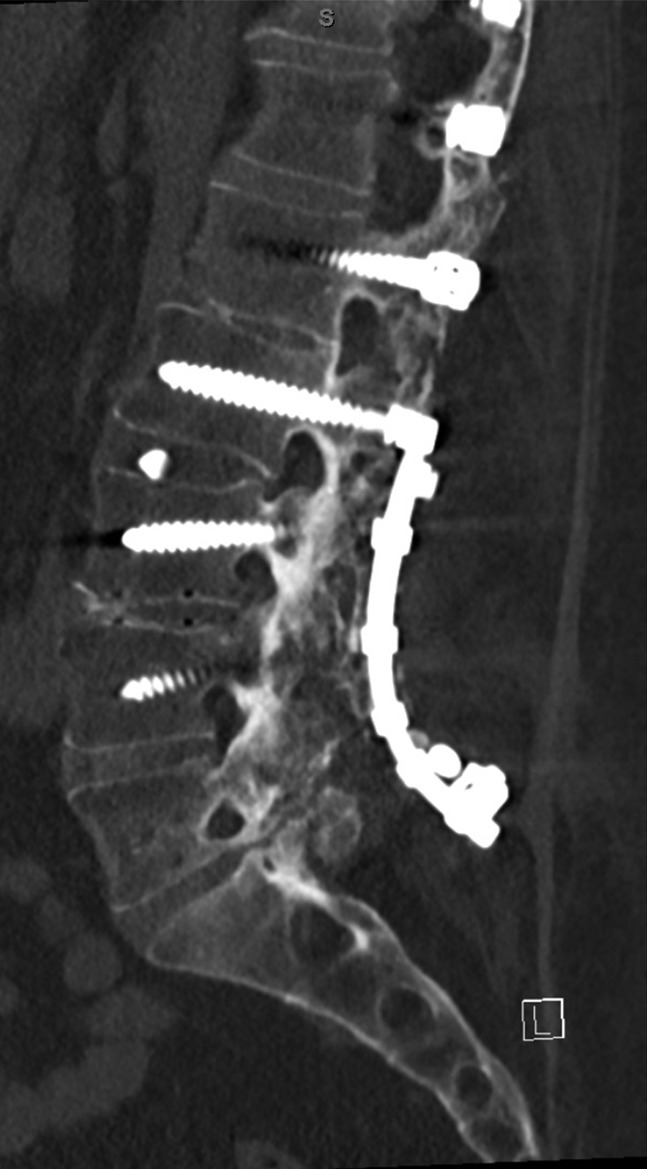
Sagittal CT lumbar spine. Demonstrating bony incorporation across the disk space and posterior elements of lumbosacral spine.
